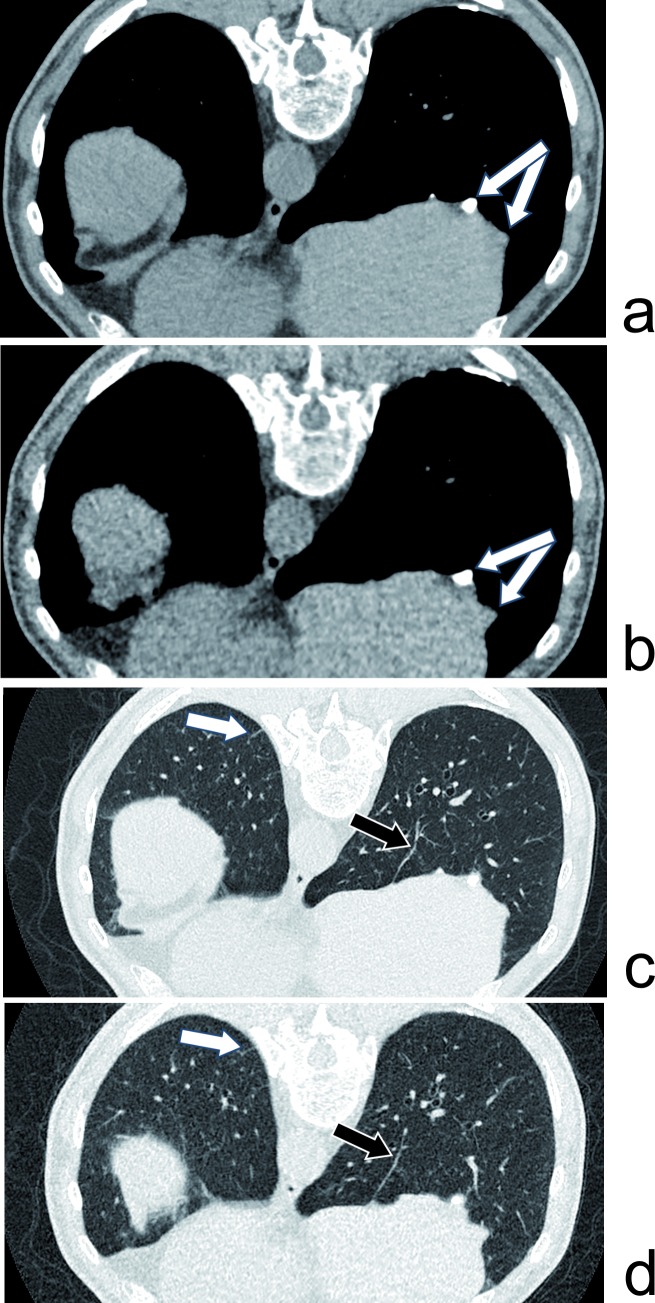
Asbestosis. 53yo male with 23 years of asbestos exposure. Pleural plaques (long white arrows), diffuse pleural thickening (parenchymal bands–black arrows) and asbestosis (subpleural intralobular and septal lines–white arrows) are depicted with equivalent diagnostic quality in the standard acquisition (a–mediastinal window, c–lung window; DLP of 291mGy.cm) and in the ULD CT (b–mediastinal window, d–lung window; DLP of 18mGy.cm).
